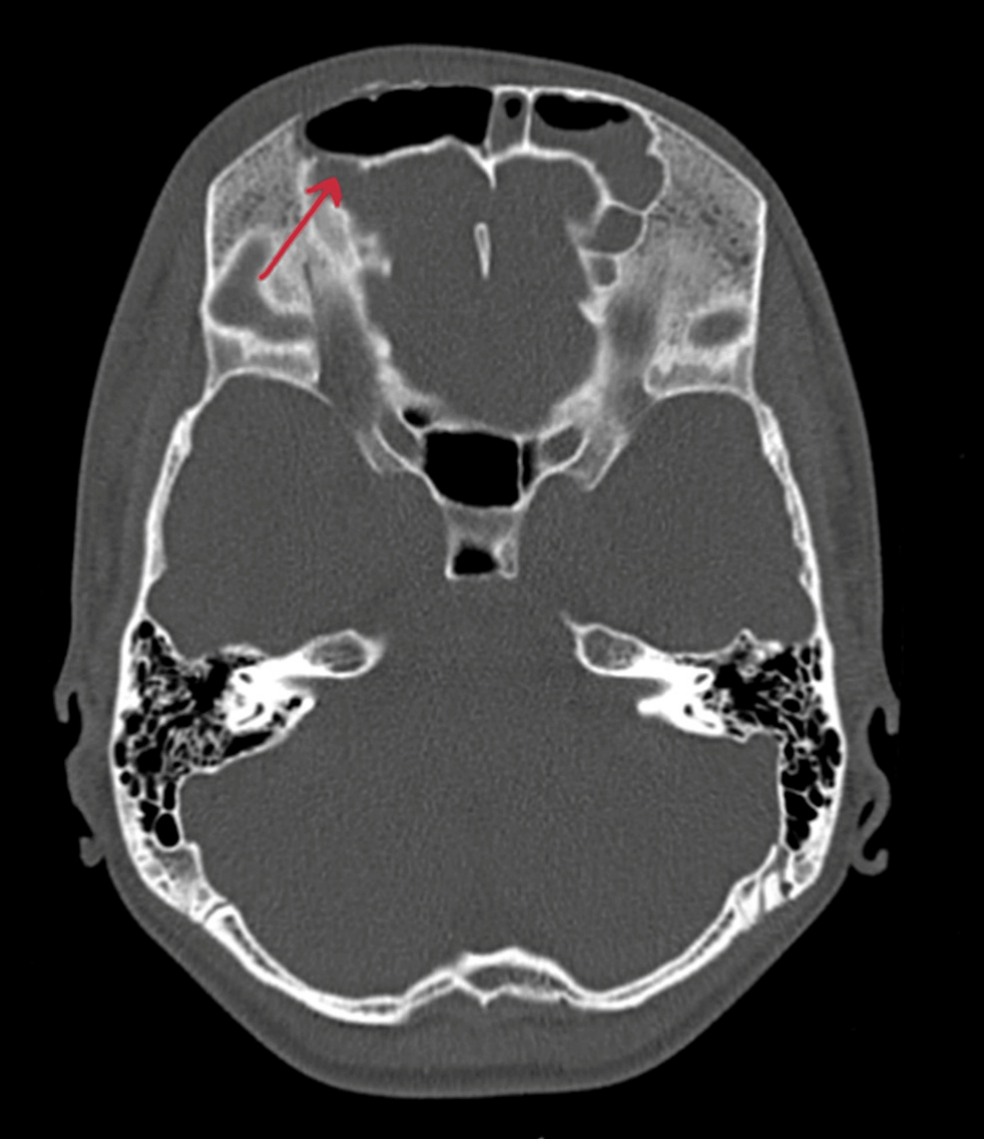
Contrast-enhanced CT showing erosion of the inner table of the frontal sinus (indicated with red arrow).
